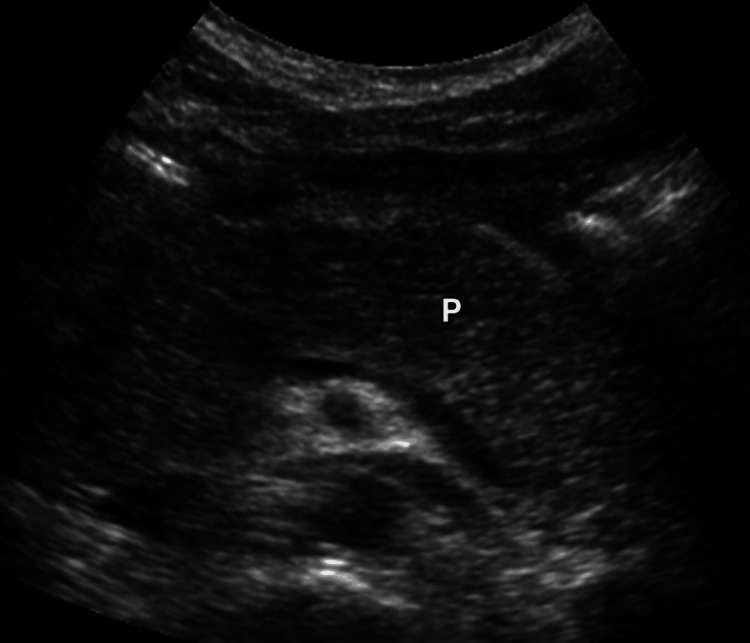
Ultrasonographic appearance of acute pancreatitis in symptomatic patients
